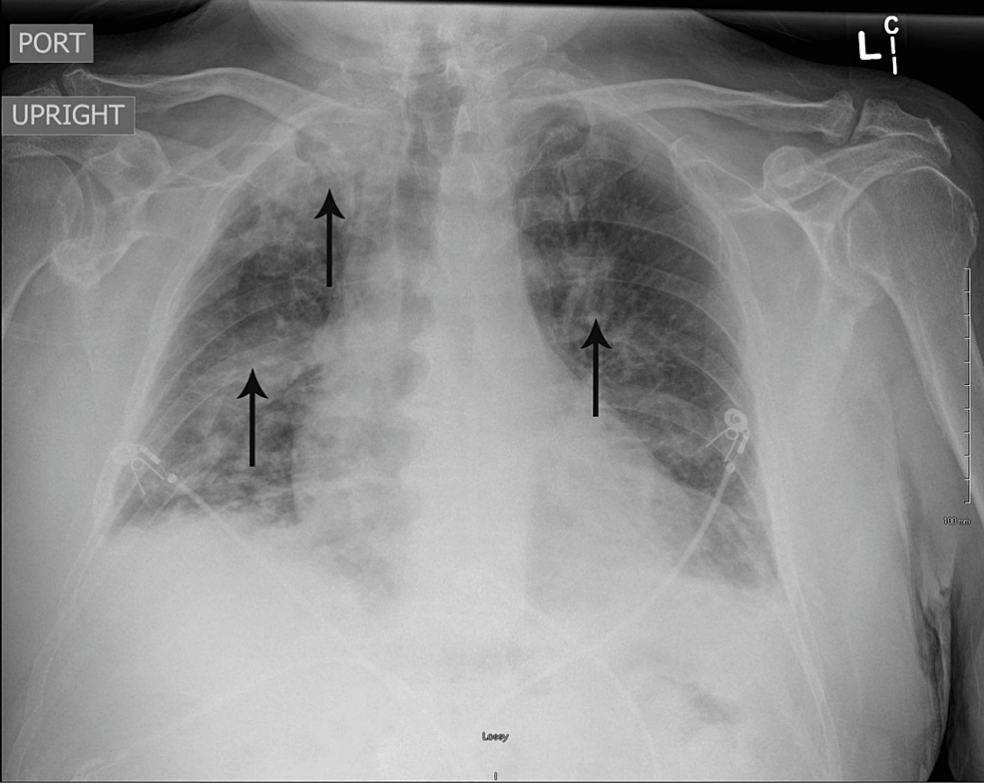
Chest X-ray showing bilateral lung opacities (arrows)
